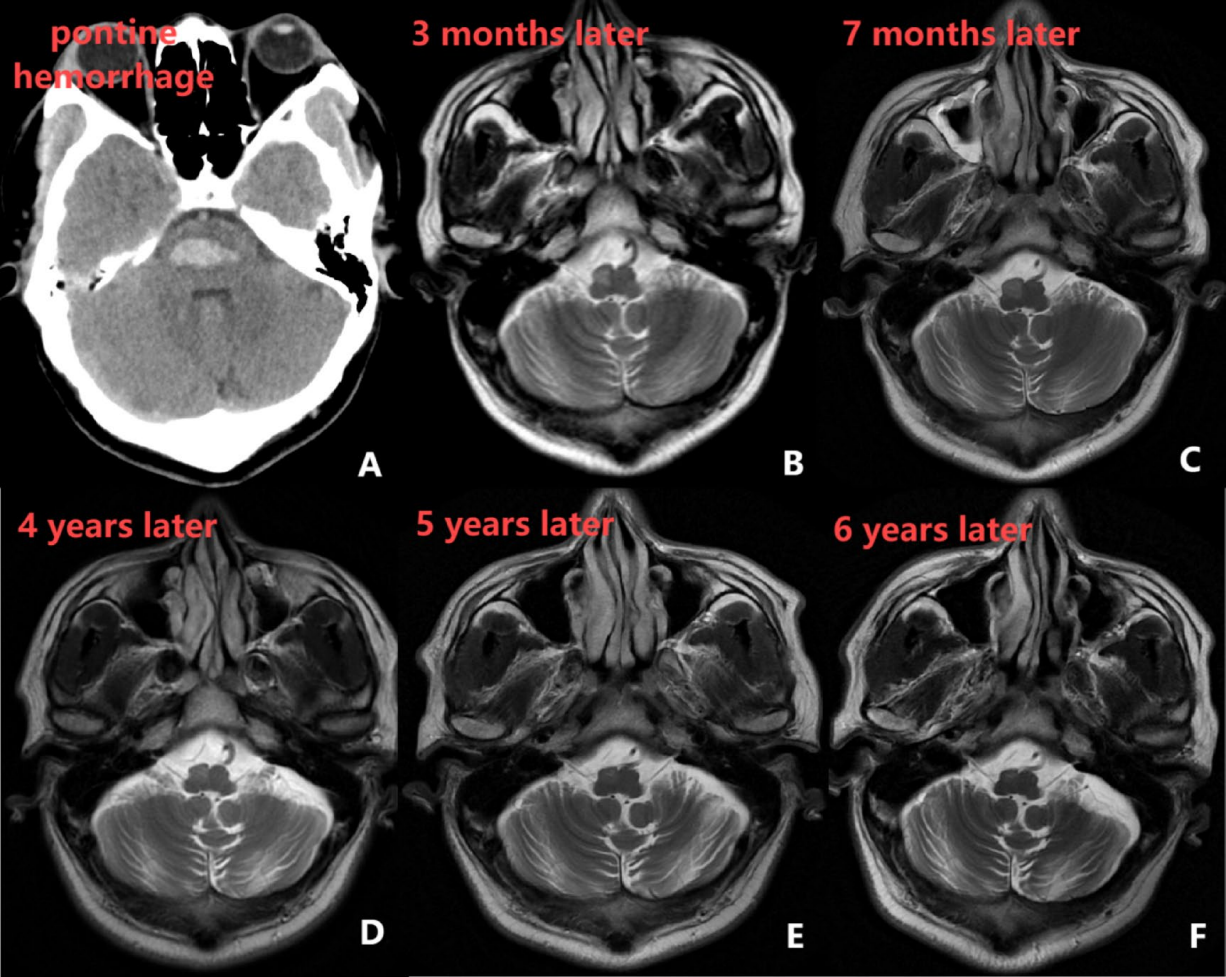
These MR image series depict the HOD dynamic process of patient 12 in Table 1 who had pontine hemorrhage (A).Within the first 3 months of insult, the ION has increased signal intensity on T2WI without hypertrophy (B); After 7 months,the ION showed hyperintensity and hypertrophy signal (C). Hypertrophy regresses gradually and only hyperintensity is seen 4 years later. Hyperintensity persists indefinitely. Over time, the olives undergoes atrophy (D–F).
